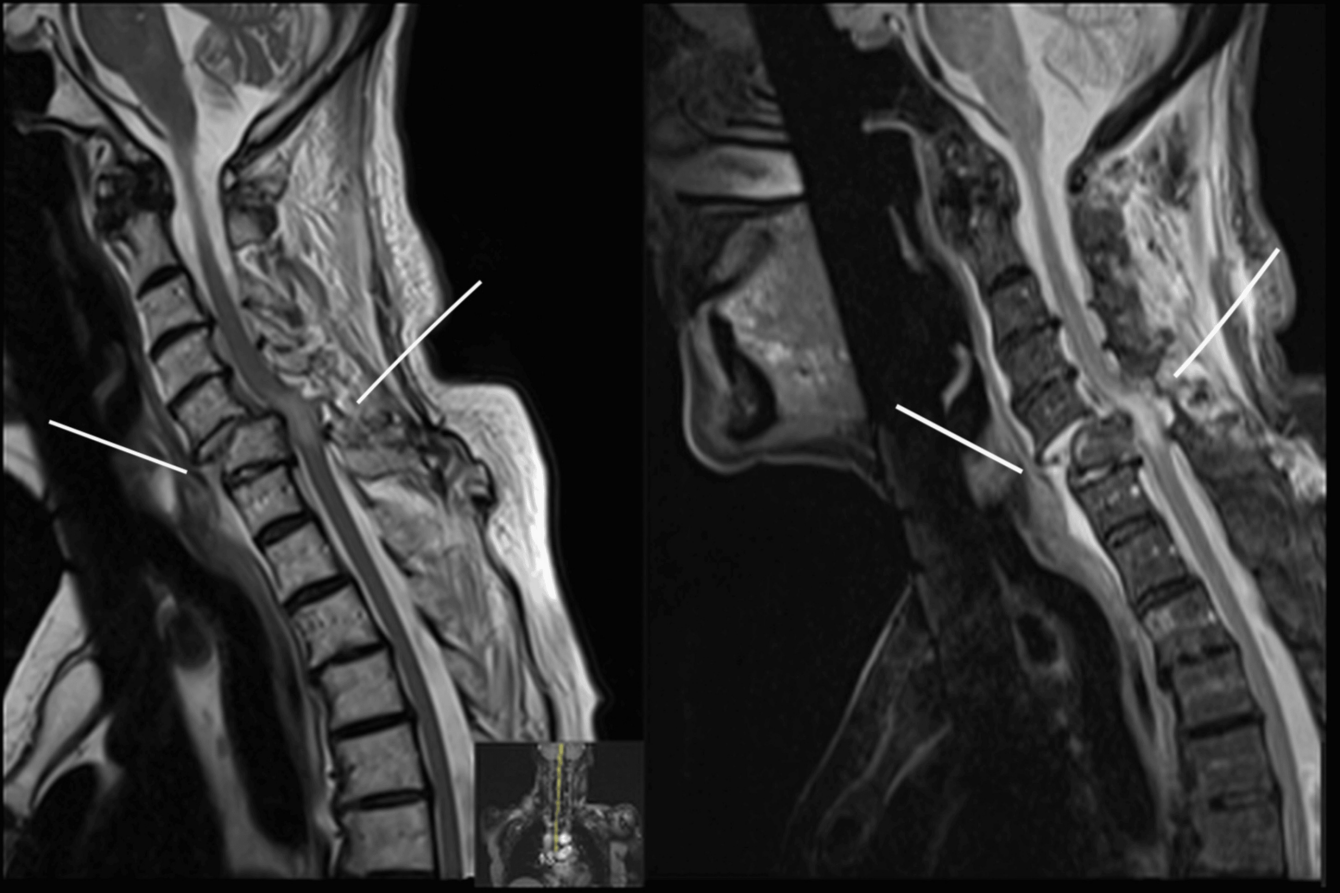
MRI images demonstrating C5-C6 dislocation, with marked anterolisthesis of C5 over C6 and dislocation of the C5-C6 facet joints. There is significant spinal cord compression at this level, with a high STIR signal within the cord. Abnormally high STIR signal is also seen in the prevertebral, paravertebral, and posterior paraspinal soft tissues, consistent with hemorrhage and traumatic injury. STIR: short tau inversion recovery, MRI: magnetic resonance imaging, C5/C6: cervical vertebrae 5/6
